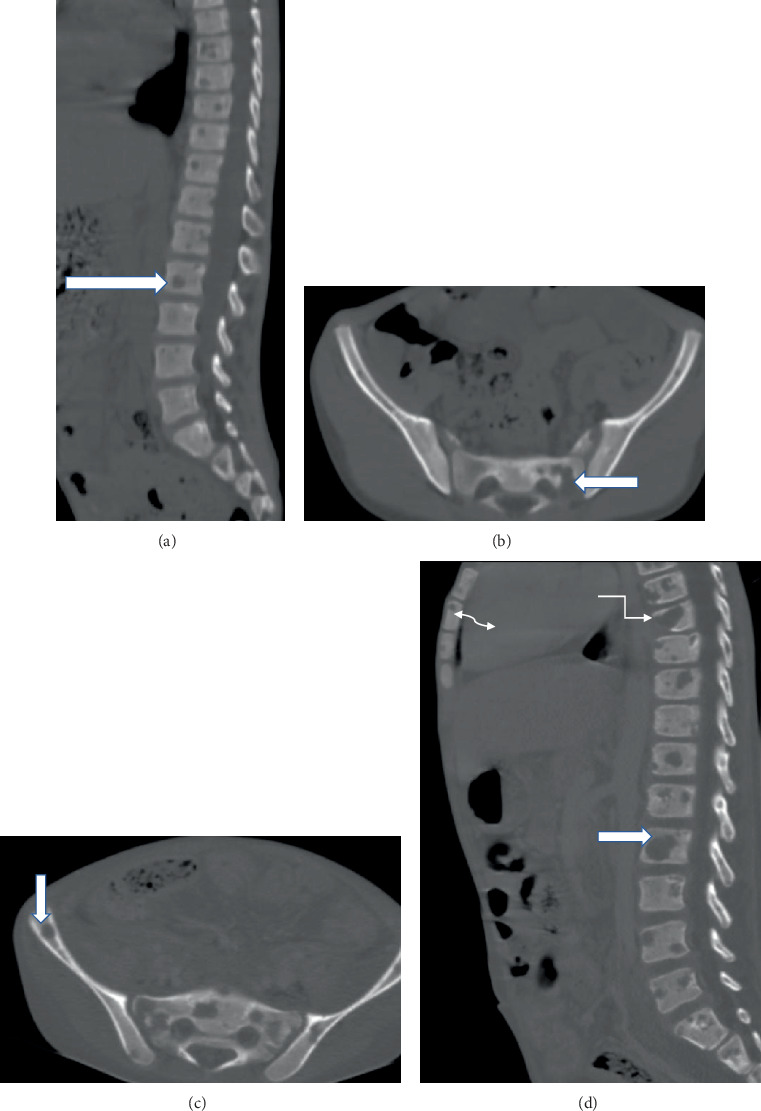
(a, b) There are multiple lytic lesions involving the lower thoracic, all lumbar, and sacral vertebrae, as well as the iliac bones, on thoracolumbar and pelvic CT (bone window) (indicated by horizontal and vertical fat arrows) taken on the first visit. (c, d) Taken after 2 months, they show an increase in the number and size of the lytic lesions and the development of a pathologic wedge compression fracture of the Th8 vertebra (elbow arrow) and a lesion on the included sternum.
